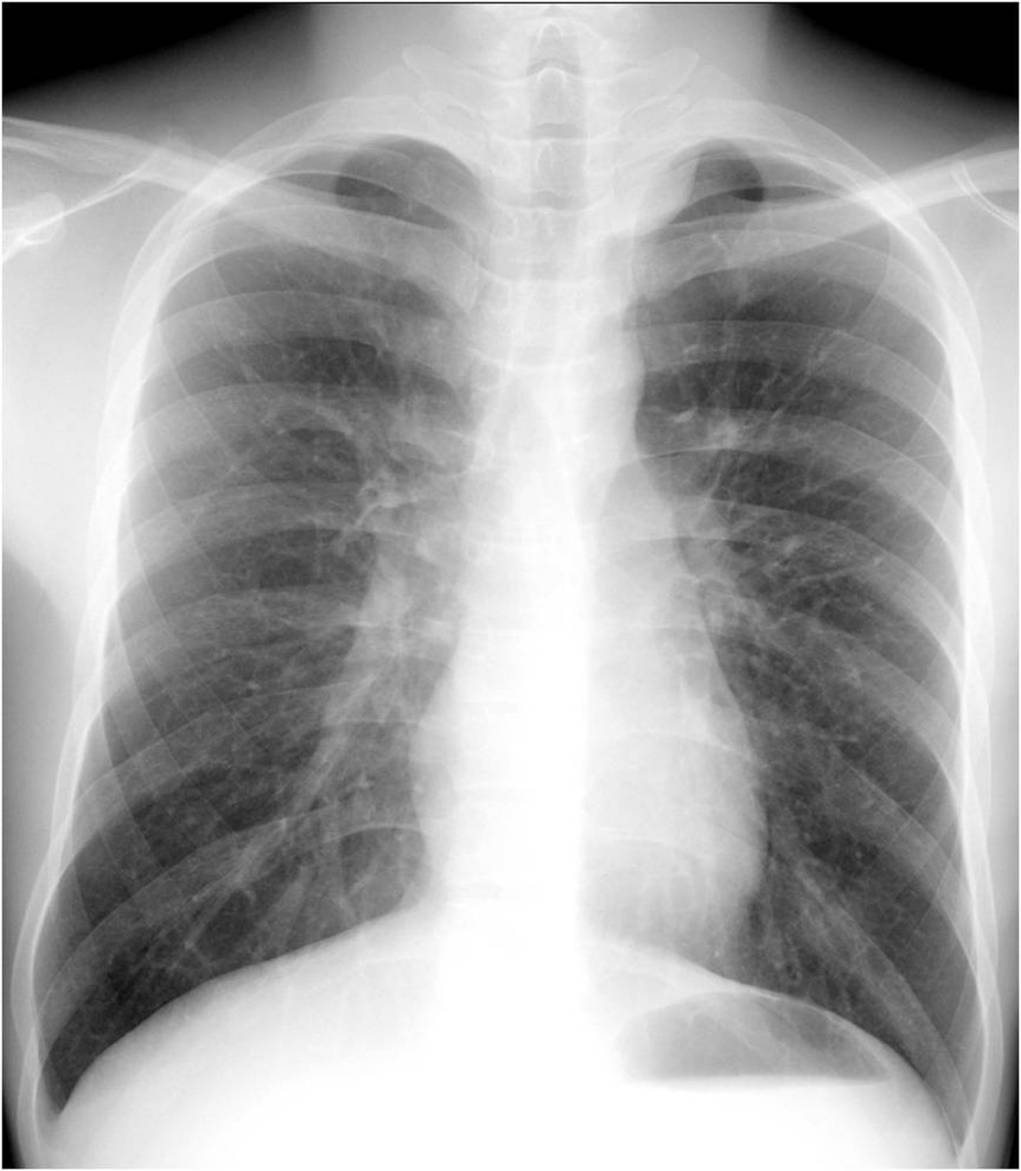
Chest radiograph showing an abnormal shadow in the superior mediastinum.
